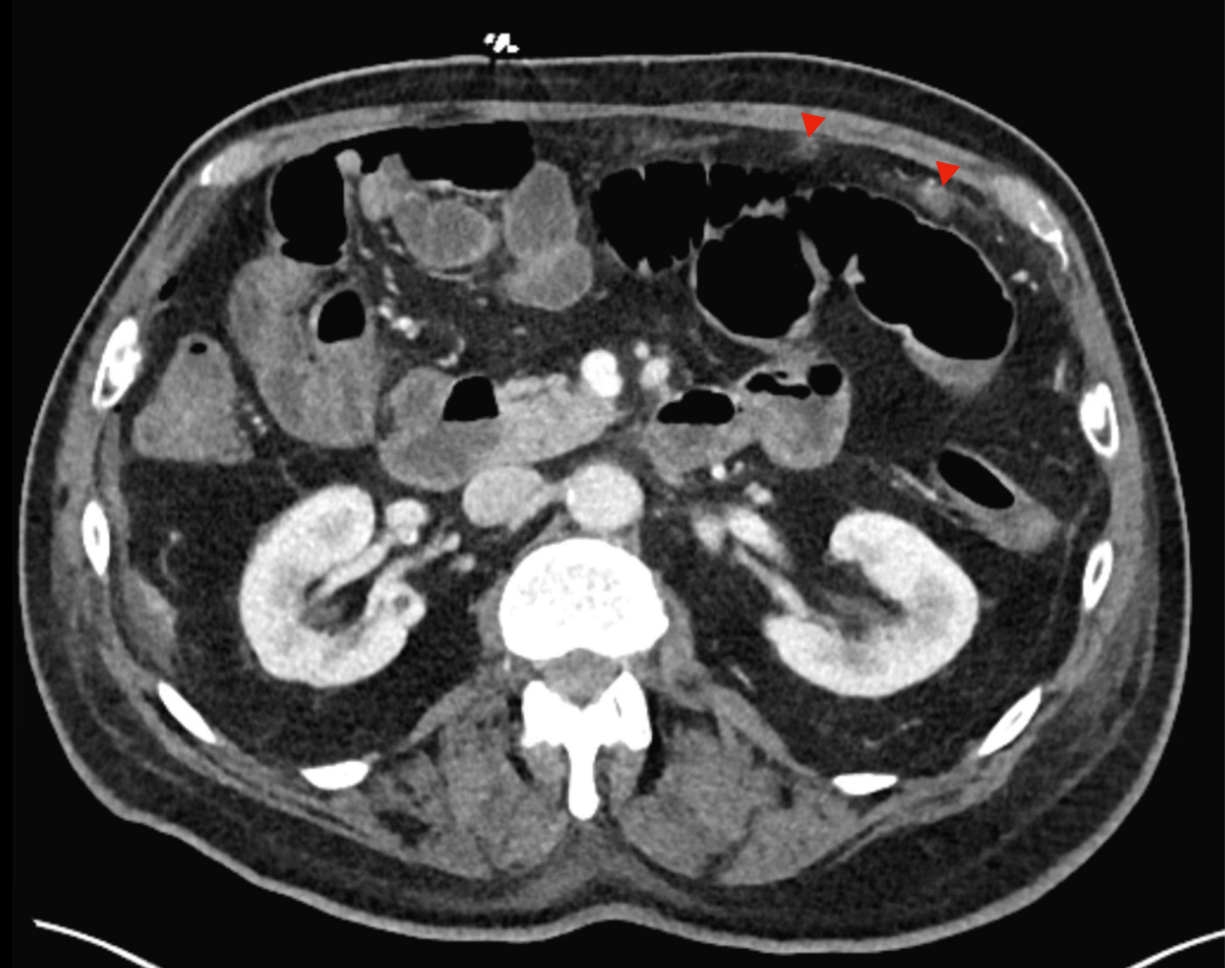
Post-operative computed tomography (CT) imaging (obtained with intravenous contrast) revealed multiple omental nodules which further reinforced the operative findings of diffuse peritoneal carcinomatosis. The red arrows highlight two separate omental nodules.
